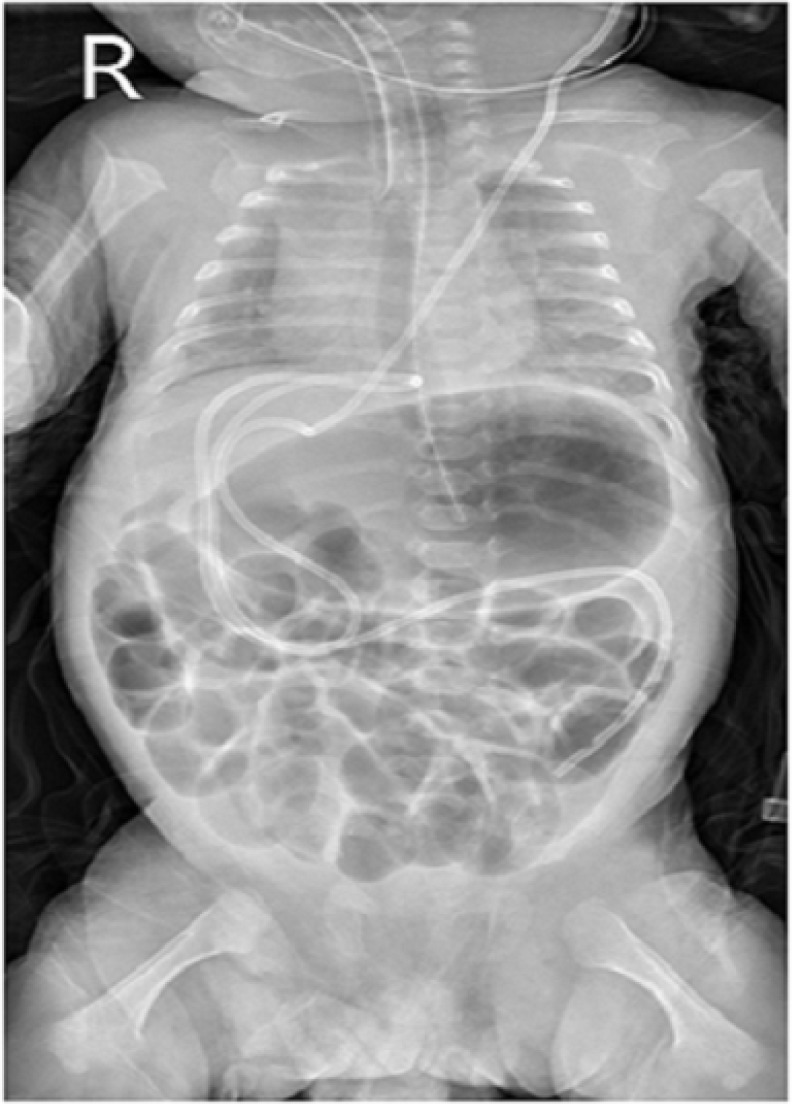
Abdominal radiography of patients after ventriculo-peritoneal shunt placement
